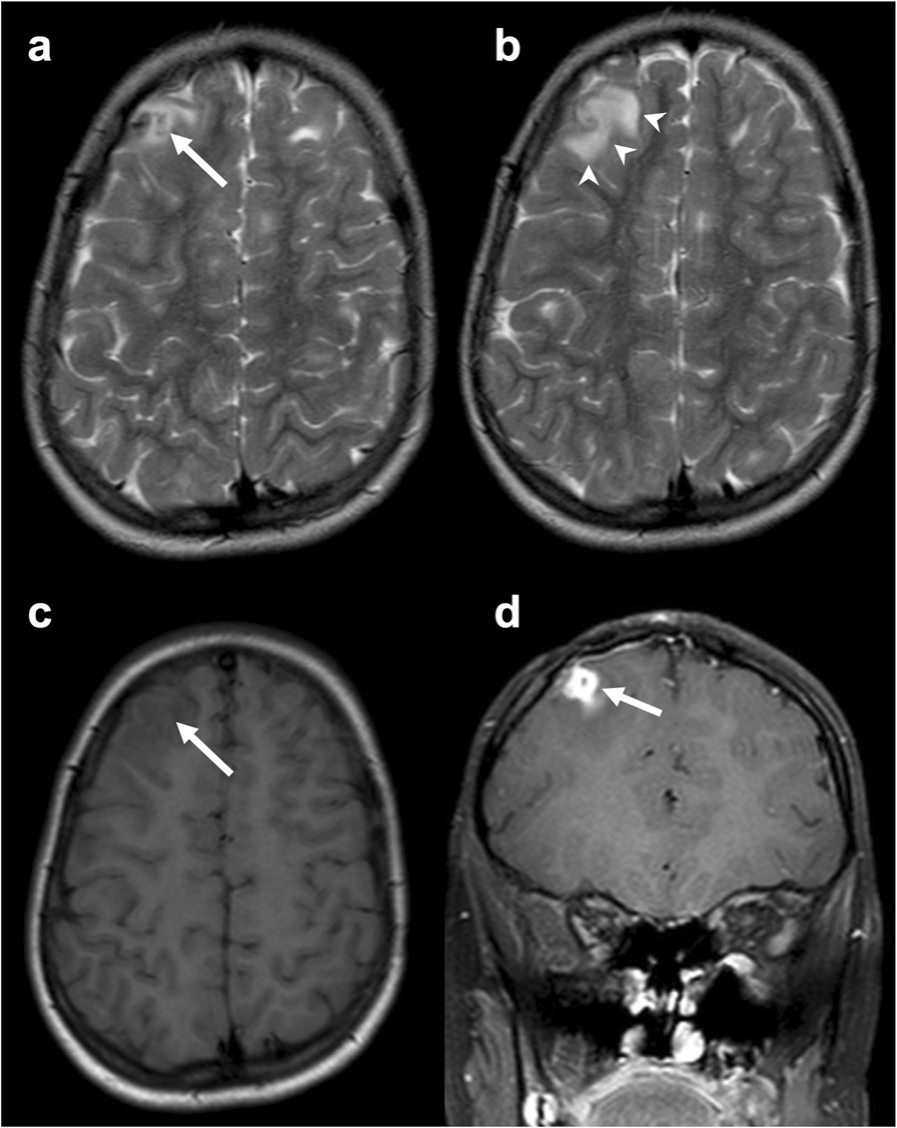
Suture material-induced granuloma. Axial T2-weighted (a, b) and unenhanced T1-weighted (c) images and coronal contrast-enhanced T1-weighted (d) showing a mass (arrow) in the right frontal superior gyrus with low signal on both T1- and T2-weighted sequences, surrounded by vasogenic edema (arrowheads), with rim enhancement. Some tubers are detectable in the axial images as the patient was affected by tuberous sclerosis
